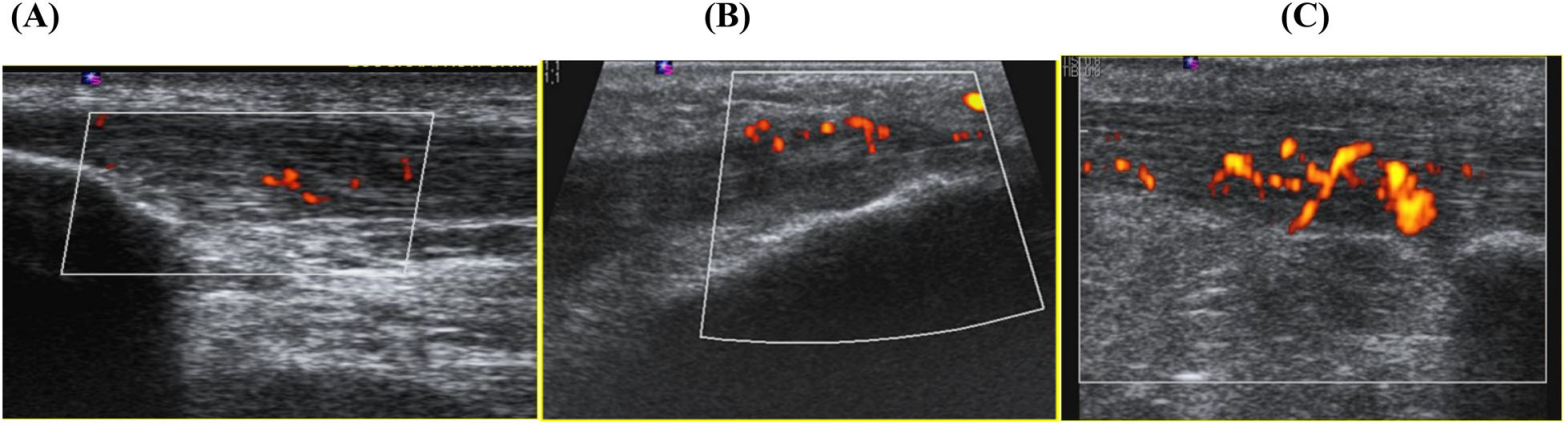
Signs of hyperemia on power‐color Doppler: (A) grade 1–1 to 2 new vessels, (B) grade 2—few vessels/low blood flow, and (C) grade 3—many vessels/considerable blood flow.
